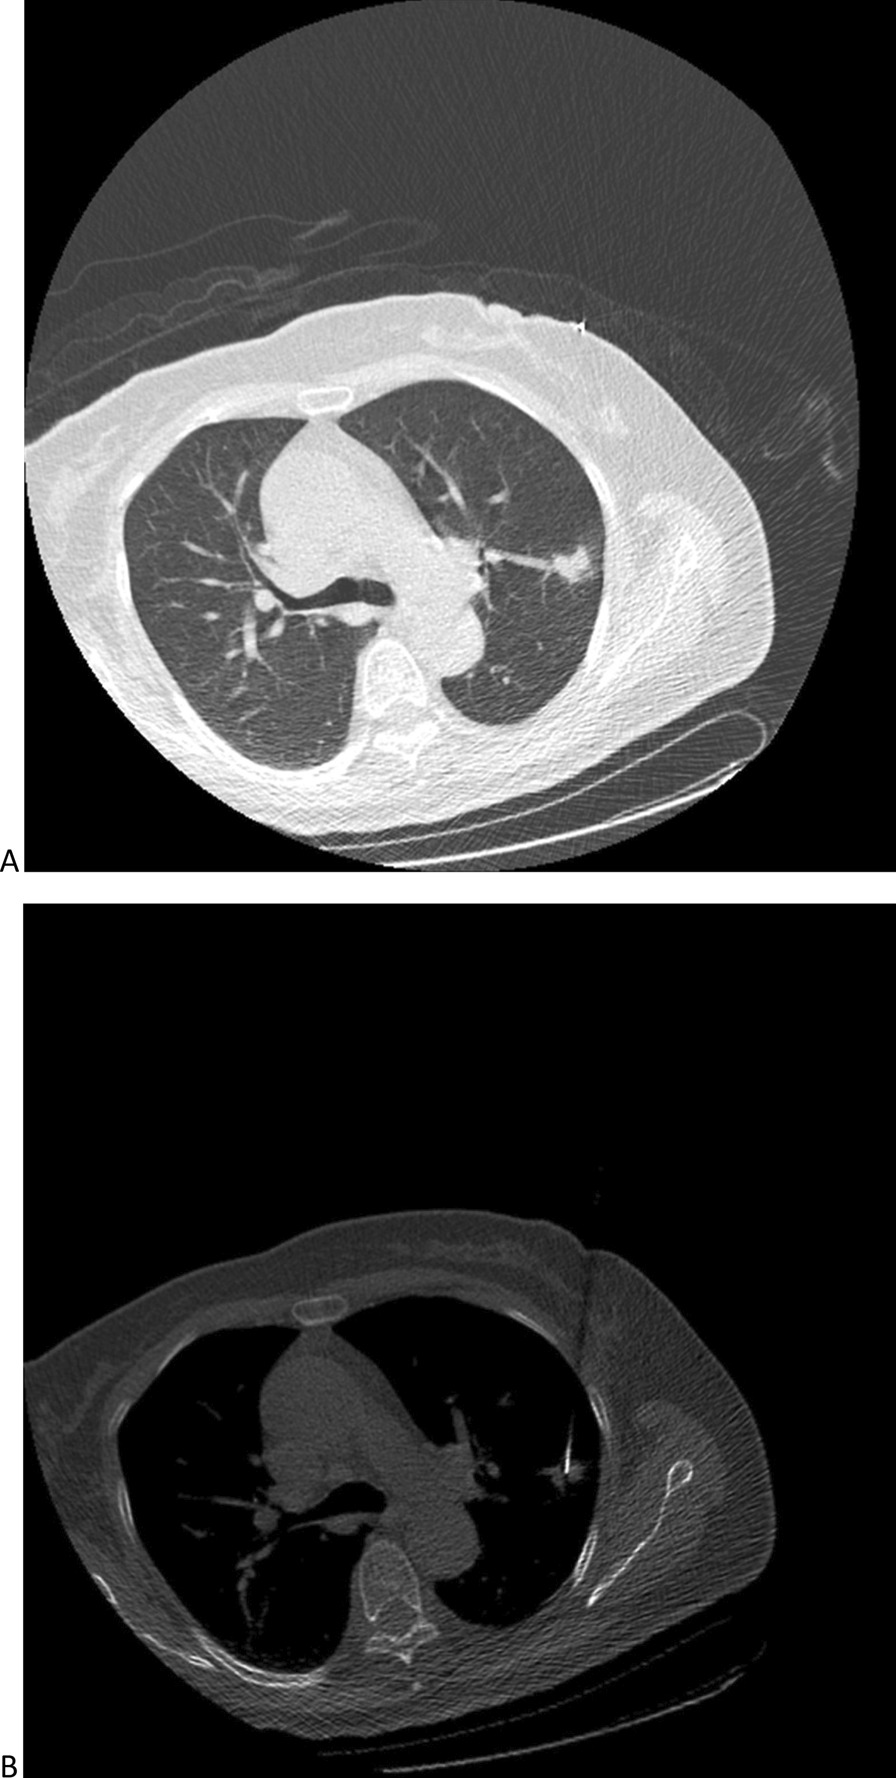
The images for low-dose CT-guided biopsy for PN. a Preoperative CT for the PN; b the procedure of low-dose CT-guided biopsy
